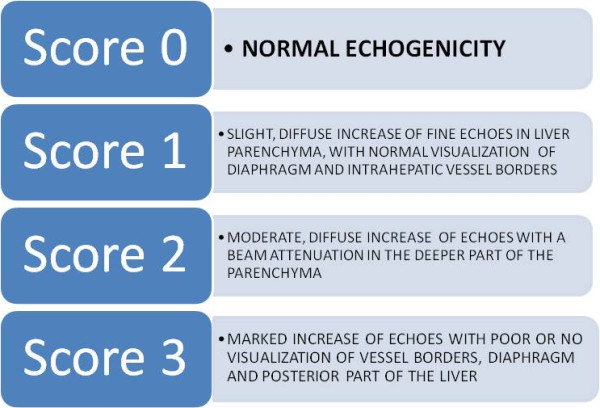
Bright liver score. The severity of steatosis can be evaluated by ultrasonography as fatty infiltration in the liver produces a diffuse increase in echogenicity.
